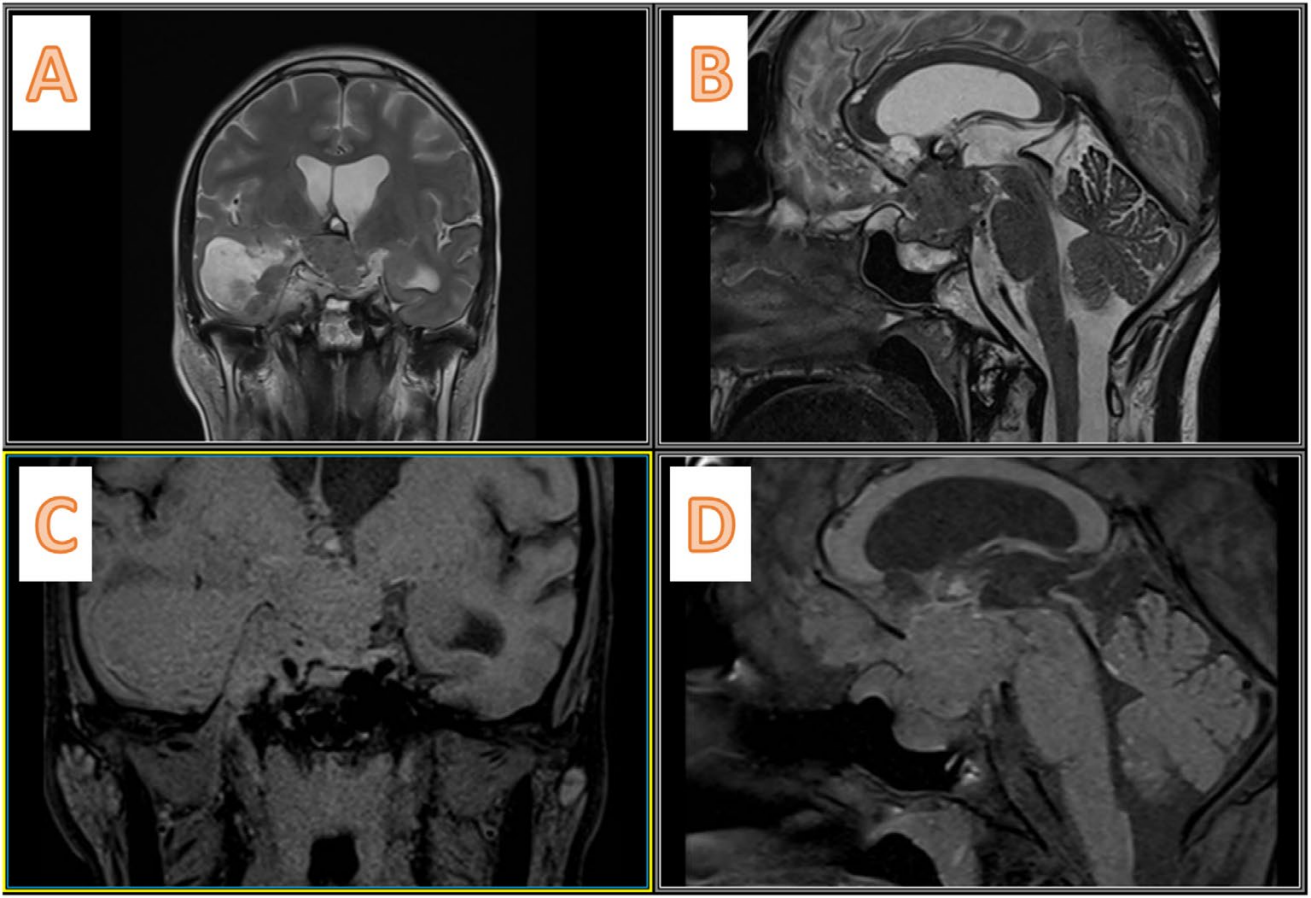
Preoperative non‐contrast enhanced MRI. (A) T2 weighted coronal image depicts the solid cystic lesion extending from sella turcica to the right middle fossa and abutting the Meckel's cave. (B) T2 weighted sagittal image shows iso to hyperintense mass within the sella cavity with extension to the third ventricle floor and compressive effect on the foramen of the Monro, causing biventricular hydrocephalus. (C, D) T1 weighted coronal and sagittal images indicate iso to hyperintense lesion.
